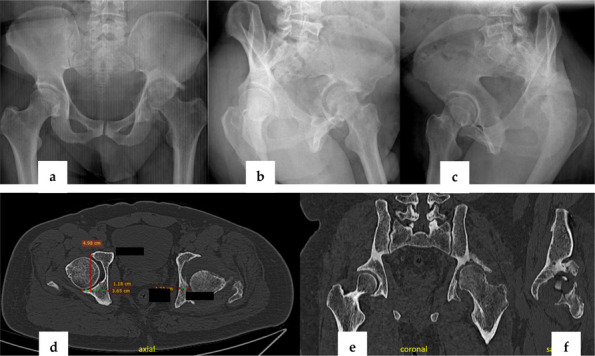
Pre-operative Diagnostic Imaging: a pelvis anteroposterior radiograph, b pelvis right obturator Judet radiograph; c pelvis right iliac Judet radiograph, d axial cut of plain pelvis computed tomography scan; e coronal cut of plain pelvis computed tomography scan; f sagittal cut of plain right hip computed tomography scan
